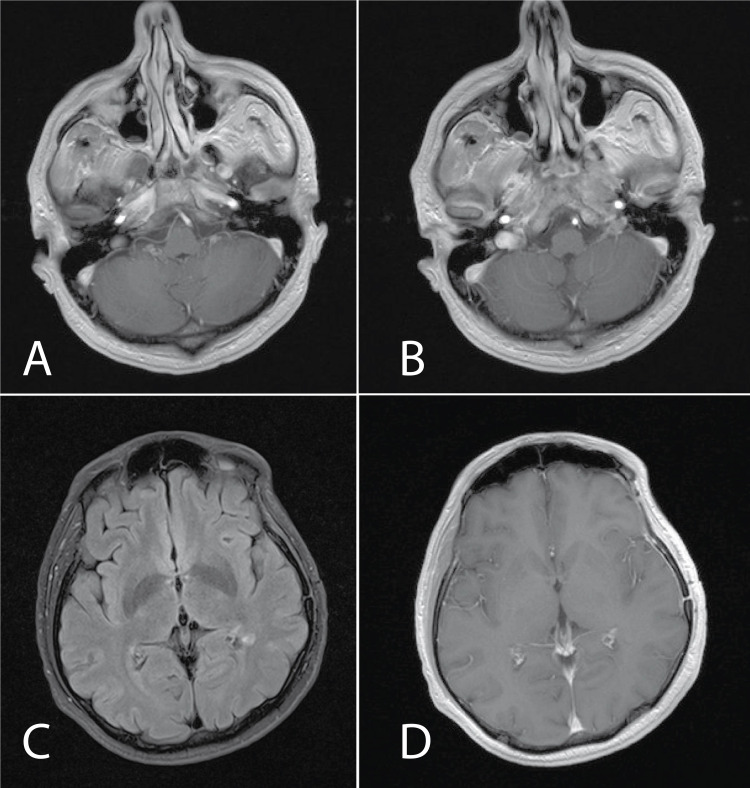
Brain MRI with IVC after treatment with steroids. A. complete resolution of lesion with open ring-type non-homogeneous contrast enhancement in cerebellum (T1 post-contrast sequence); B. complete resolution of hyperintense lesions in cerebellum (T1 post-contrast sequence); C. resolution of left periventricular lesion (T2/FLAIR sequence); D. resolution of left periventricular lesion (T1 post-contrast sequence). IVC: intravenous contrast; FLAIR: fluid-attenuated inversion recovery
